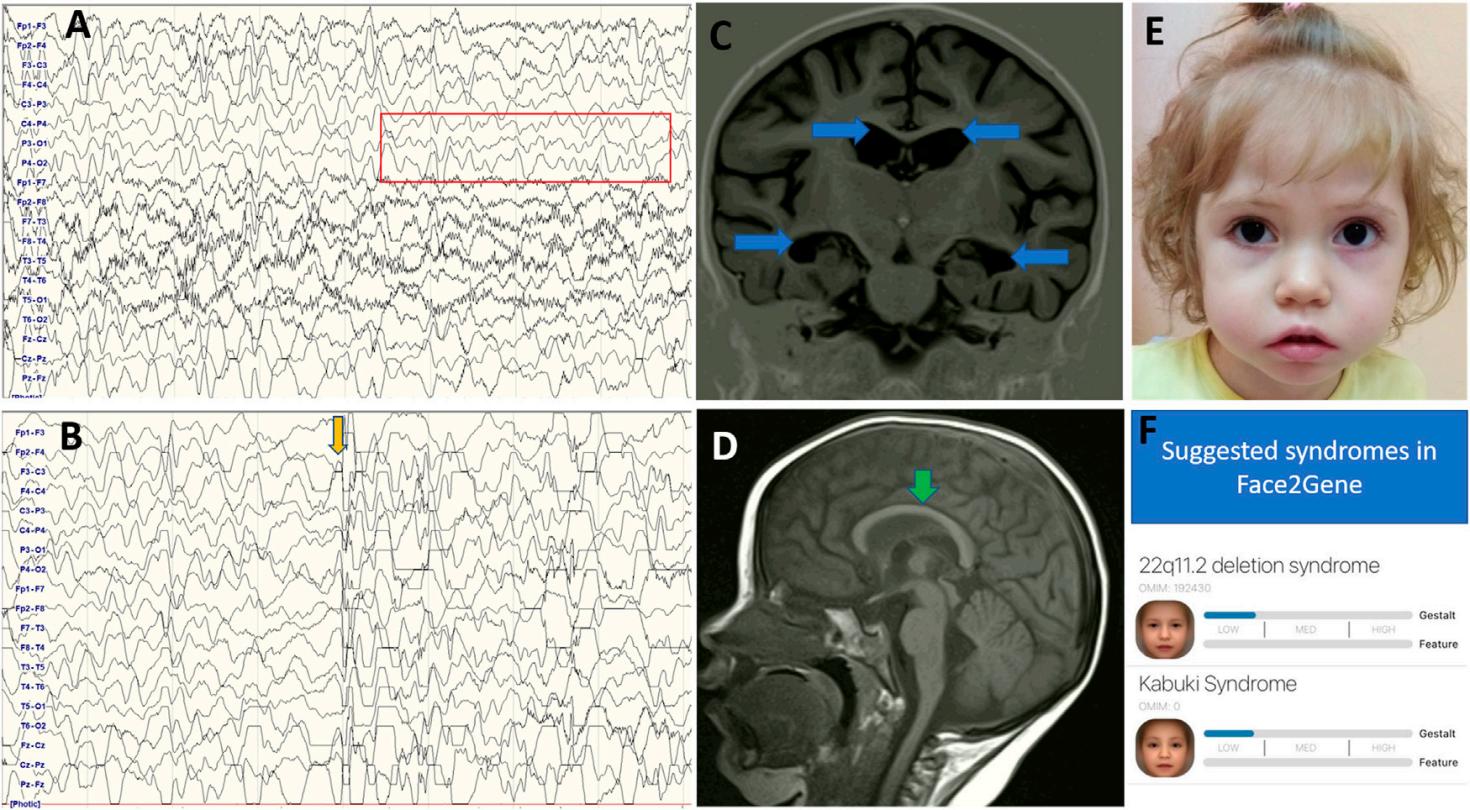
EEG longitudinal bipolar montage. Sweep: 30 mm/s; sensitivity: 150 mV/mm; bandpass: 1–70 Hz. Awake EEG (A) showing slow background activity with normal posterior dominant rhythm. Ictal EEG recording (B) showing myoclonic jerks with diffuse discharge and bursts of slow waves. 1.5T axial (C) and sagittal (D) T1 MRI indicated a mild diffuse brain atrophy, bilateral ventriculomegaly (blue arrows), and thinning of the corpus callosum (green arrows). Facial features (E) including a pronounced double curve of the upper lip (Cupid’s bow) and long palpebral fissures. Face2Gene analysis (F) showing low overlap with Kabuki syndrome.
